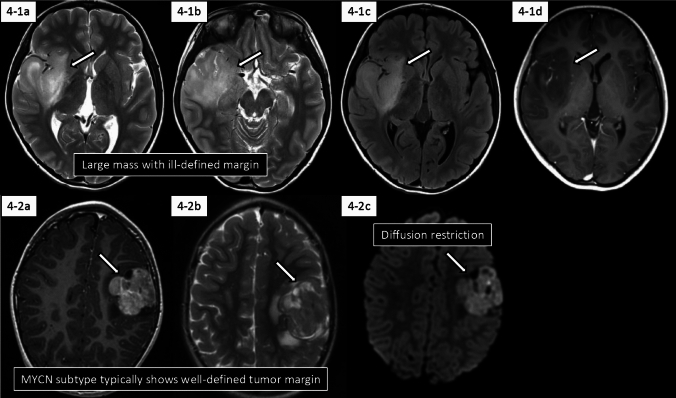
Imaging features of diffuse pediatric-type high-grade glioma, H3-wild type and IDH-wild type. 4-1 (8 years old, boy; RTK2A subclass): There is a large tumor mass with an ill-defined margin involving the right insula and temporal lobe (arrows). The tumor mass shows hyperintensity on T2-weighted imaging (4-1a and 4-1b) and fluid-attenuated inversion recovery imaging (4-1c) with a partial contrast enhancement (4-1d). 4-2 (3 years old, boy; MYCN subtype): A solid lobulated tumor mass with a well-defined margin is observed in the left frontal lobe (arrows). The tumor mass shows heterogeneous contrast enhancement on contrast-enhanced T1-weighted imaging (4-2a), hyperintensity with partial cystic components on T2-weighted imaging (4-2b), and hyperintensity on diffusion-weighted imaging (4-2c). The images of this case were cited from Tauziède-Espariat et al. [77] under a Creative Commons Attribution 4.0 International License
